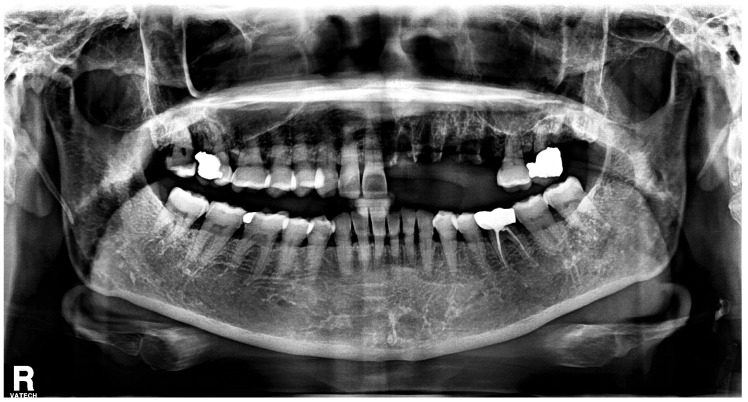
Preoperative panoramic radiograph shows multiple teeth loss and changed trabecular pattern on the left side of maxilla.
